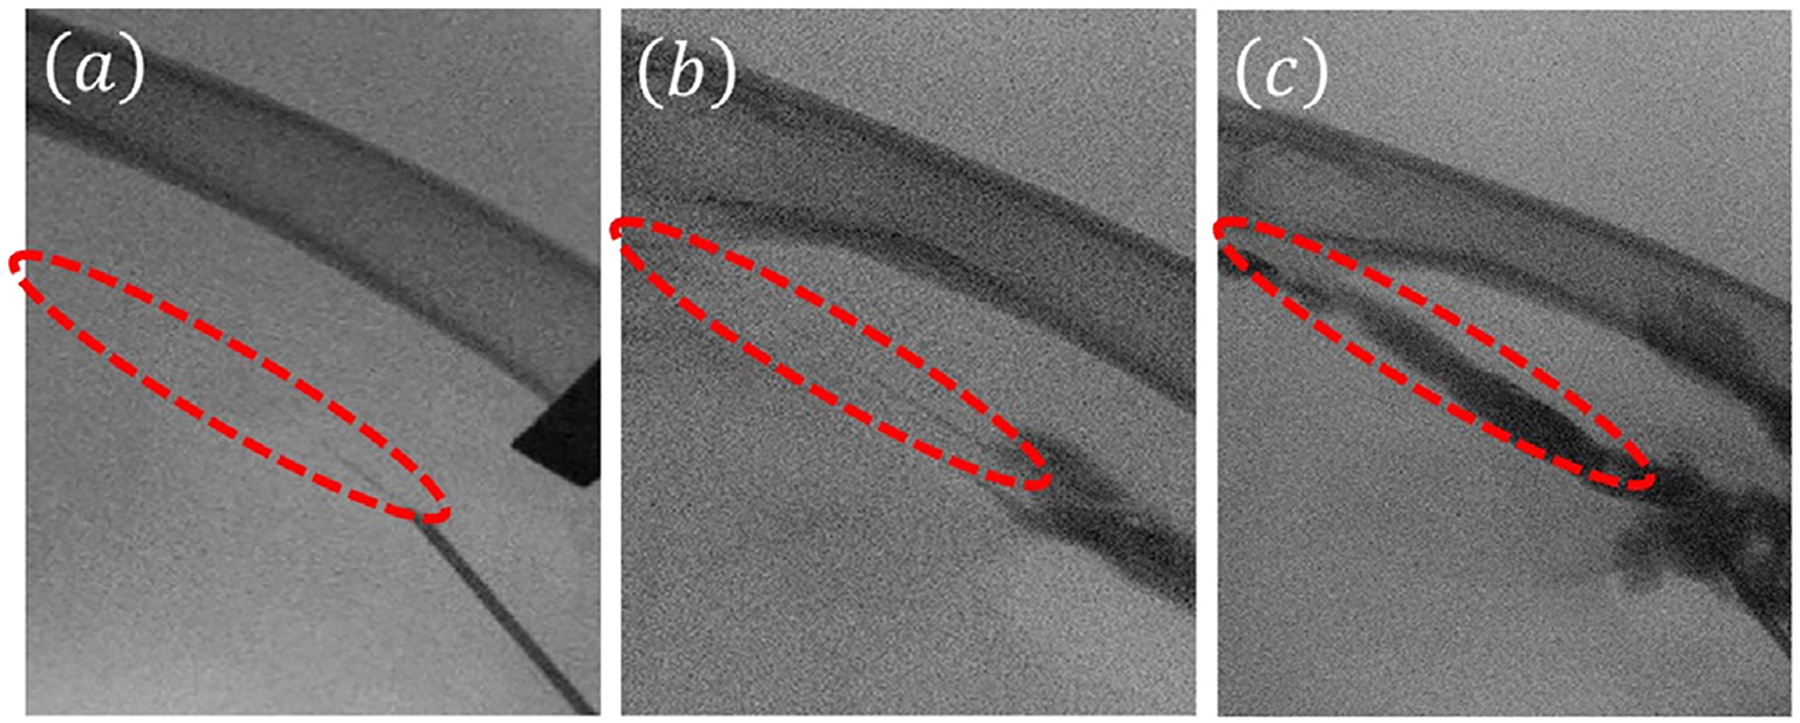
Representative C-arm angiography photographs of rabbit femoral artery atherosclerotic plaque models treated with 500 kHz FUS bursts for 15 min at 4 MPa PNP using (a) before catheter insertion, (b) after catheter insertion, and (c) after treatment using catheter-assisted FUS. Each FUS burst contained 500 cycles at 10% duty cycle with a burst repetition rate of 100 Hz. FUS, focused ultrasound; PNP, peak negative pressure.
